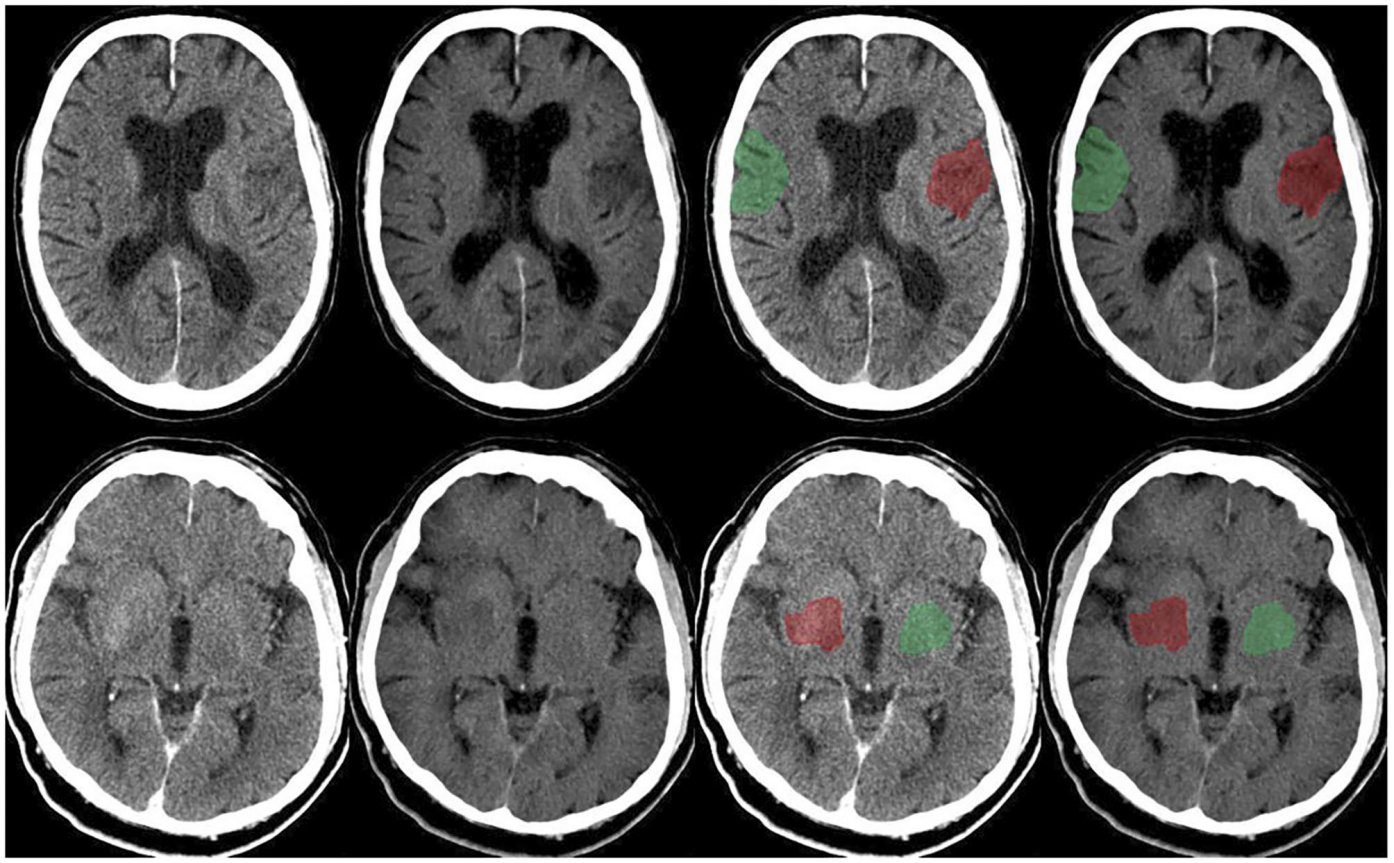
Upper row: Follow-up dual-layer dual-energy CT within 12 h in a 70-year-old man with left MCA occlusion (initial NHISS 7; ASPECTS 8) after thrombectomy (TICI 3), time onset to reperfusion 184 min. Conventional polychromatic image (first and third from the left), VNC image (second and fourth from the left). Example of ischemic ROI placement (red) and contralateral normal ROI placement (green). Lower row: Follow-up dual-layer dual-energy CT within 12 h in an 89-year-old man with right MCA occlusion (initial NHISS 17; ASPECTS 8) after thrombectomy (TICI 3), time onset to reperfusion 154 min. Conventional polychromatic image (first and third from the left), VNC image (second and fourth from the left). Example of ischemic ROI placement (red) and contralateral normal ROI placement (green).
